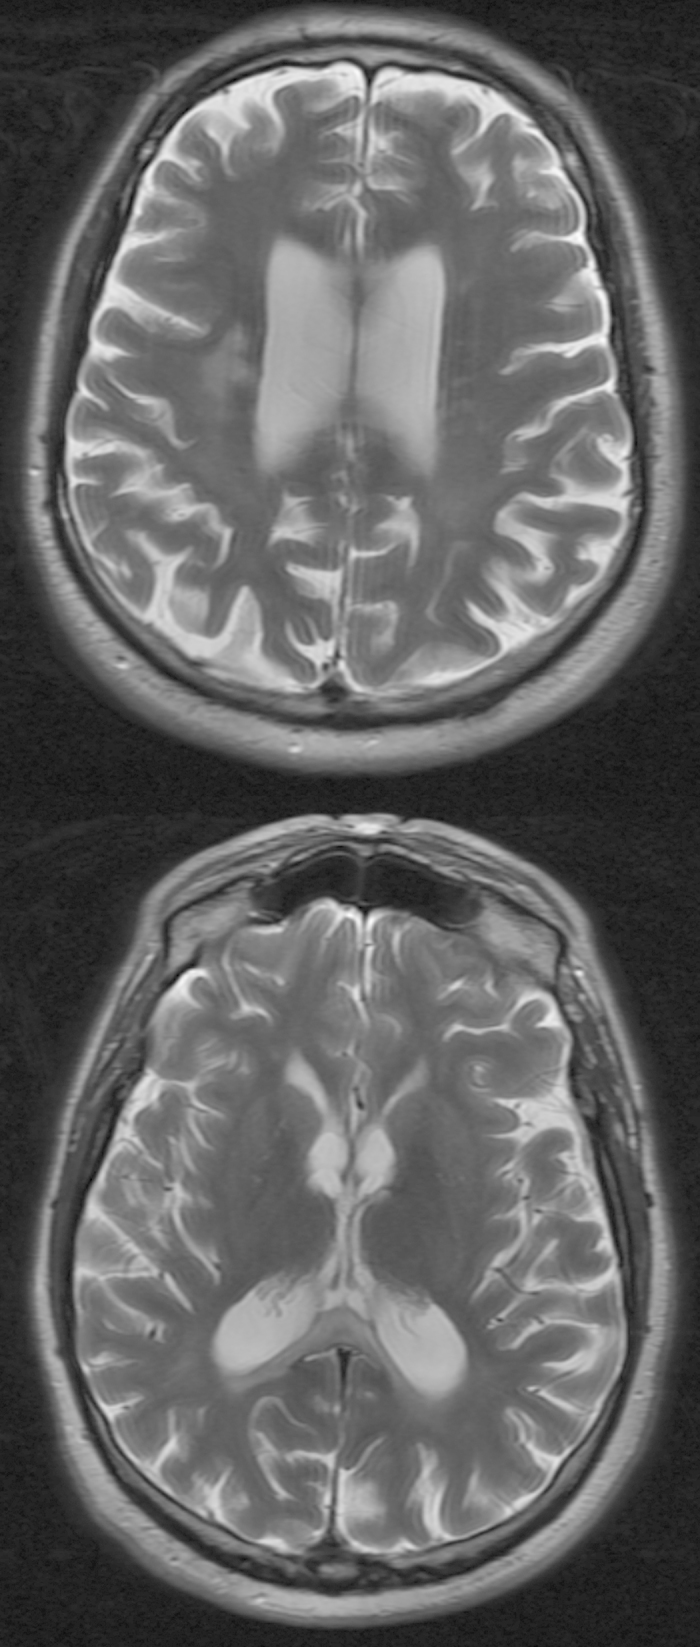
Axial T2-weighted images including the corpus callosum and peri-rolandic white matter. The MRI shows multifocal involvement of the corpus callosum with volume loss, particularly posteriorly. There is asymmetric white matter involvement with sparing of the sub-cortical u-fibres. White matter deep to the pre-central and post-central gyri is specifically involved. No signal change is found within the pyramidal tracts more inferiorly. In places there is apparent involvement of white matter lying transverse to the long axis of the lateral ventricles. Otherwise there is mild cerebellar volume loss, mild parietal and frontal atrophy. The brainstem is normal.
